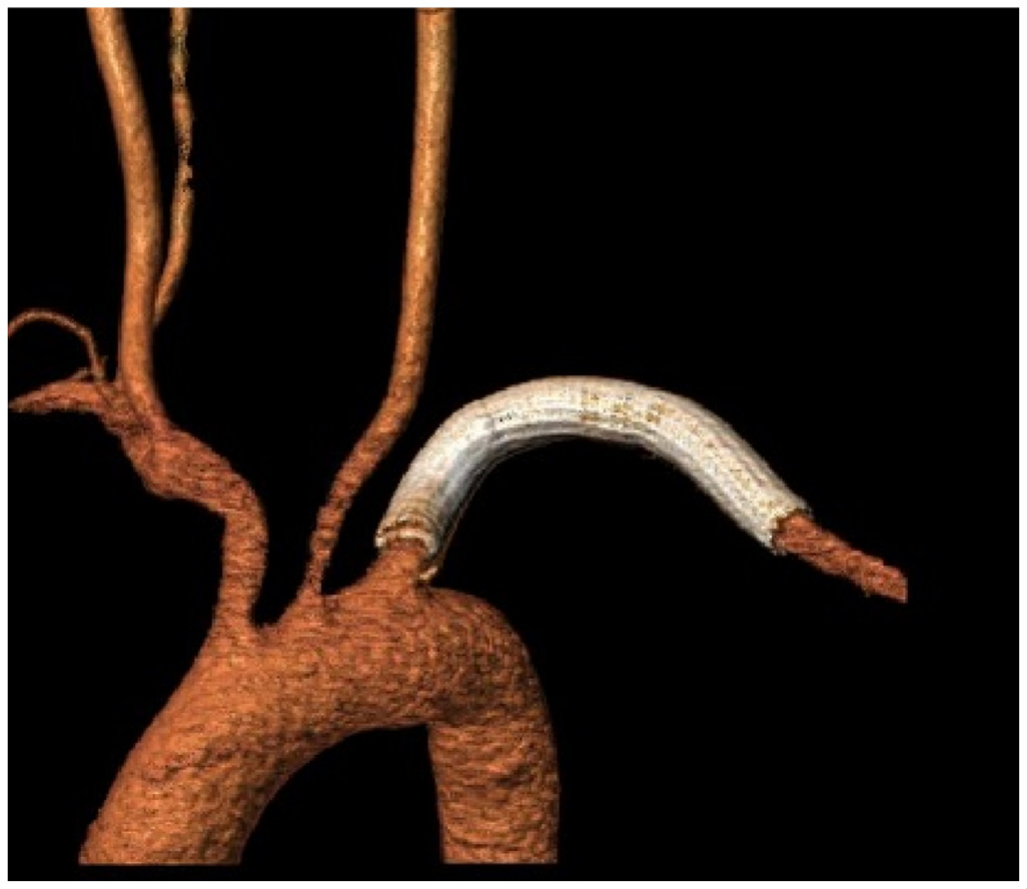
Three-dimensional reconstruction of computed tomography arteriography (CTA) image for case 1 at 1 month after the endovascular procedure, revealed normal left subclavian artery (LSA) flow.
